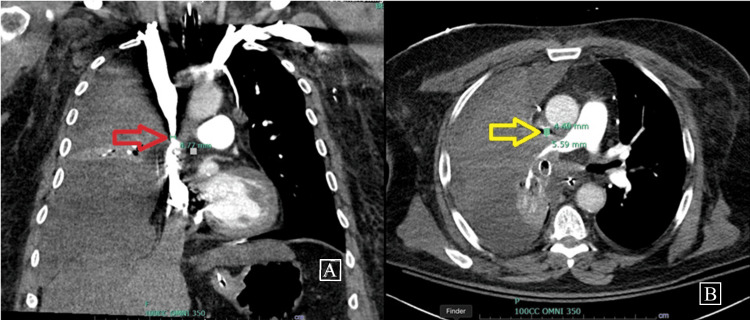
Computed tomography of the chest demonstrating compression of the SVC. The red arrow indicates the site of stenosis in the coronal plane (A), while the yellow arrow highlights the stenosis in the axial plane (B). SVC: superior vena cava
